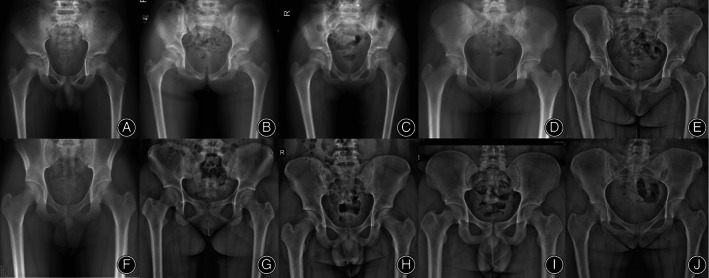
Hip radiographs of children aged 10–19 years, the femoral neck‐shaft angle decreases with the increase of age. (A): 10 years old; (B): 11 years old; (C): 12 years old; (D): 13 years old; (E): 14 years old; (F): 15 years old; (G): 16 years old; (H): 17 years old; (I): 18 years old; (J): 19 years old.
